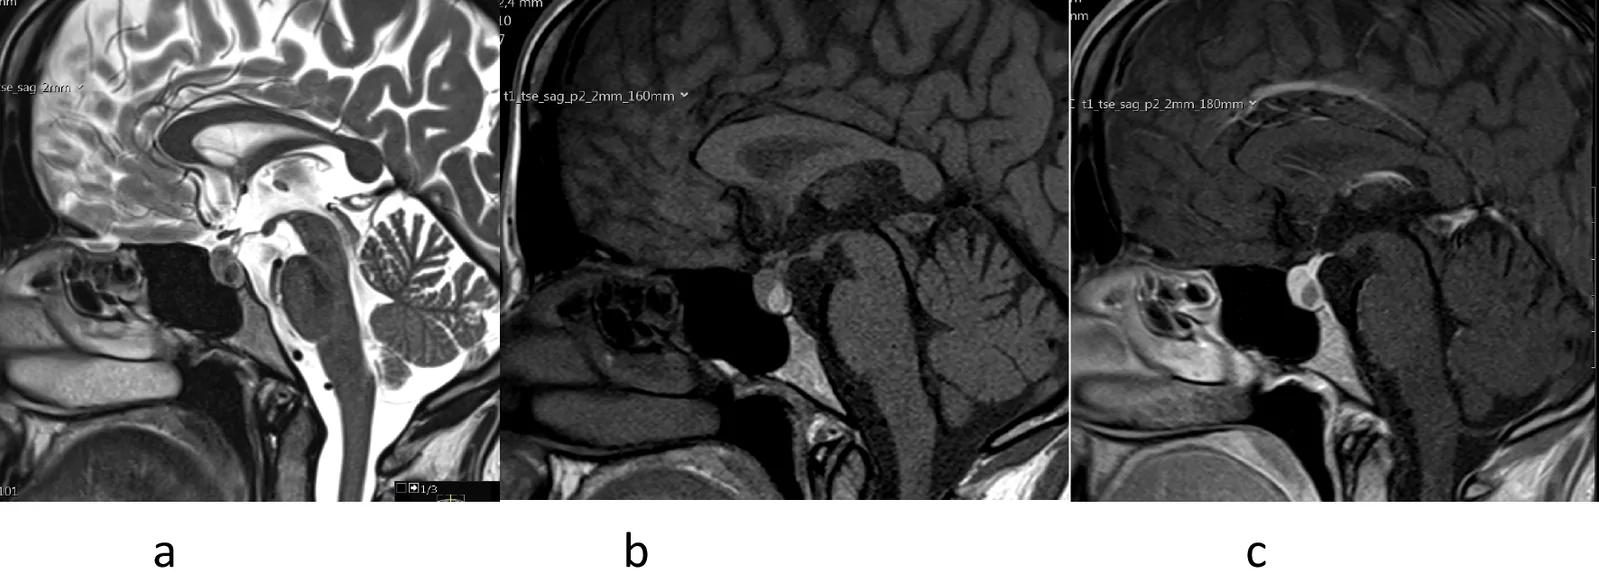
Hyperproteinic RCC. The sagittal T2-weighted image (a) demostrates a homogeneous hypointense mass located on the midline between the adenohypophysis and neurohypophysis. The sagittal T1-weighted image (b) shows the hyperintense signal of the lesion. The contrast-enhanced sagittal T1-weighted image (c) shows no enhancement of the lesion
